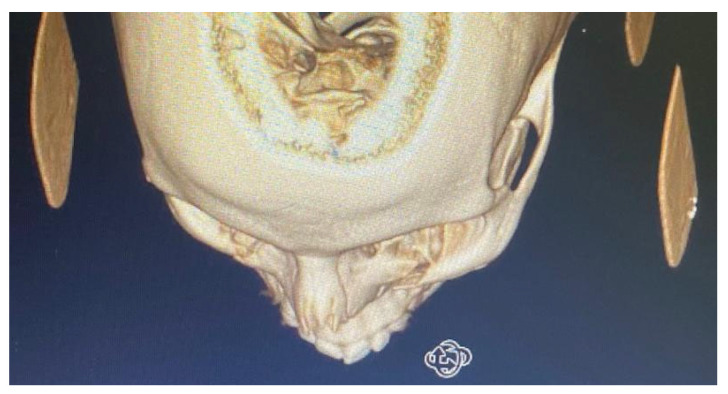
CBCT of left infraorbital rim fracture.
